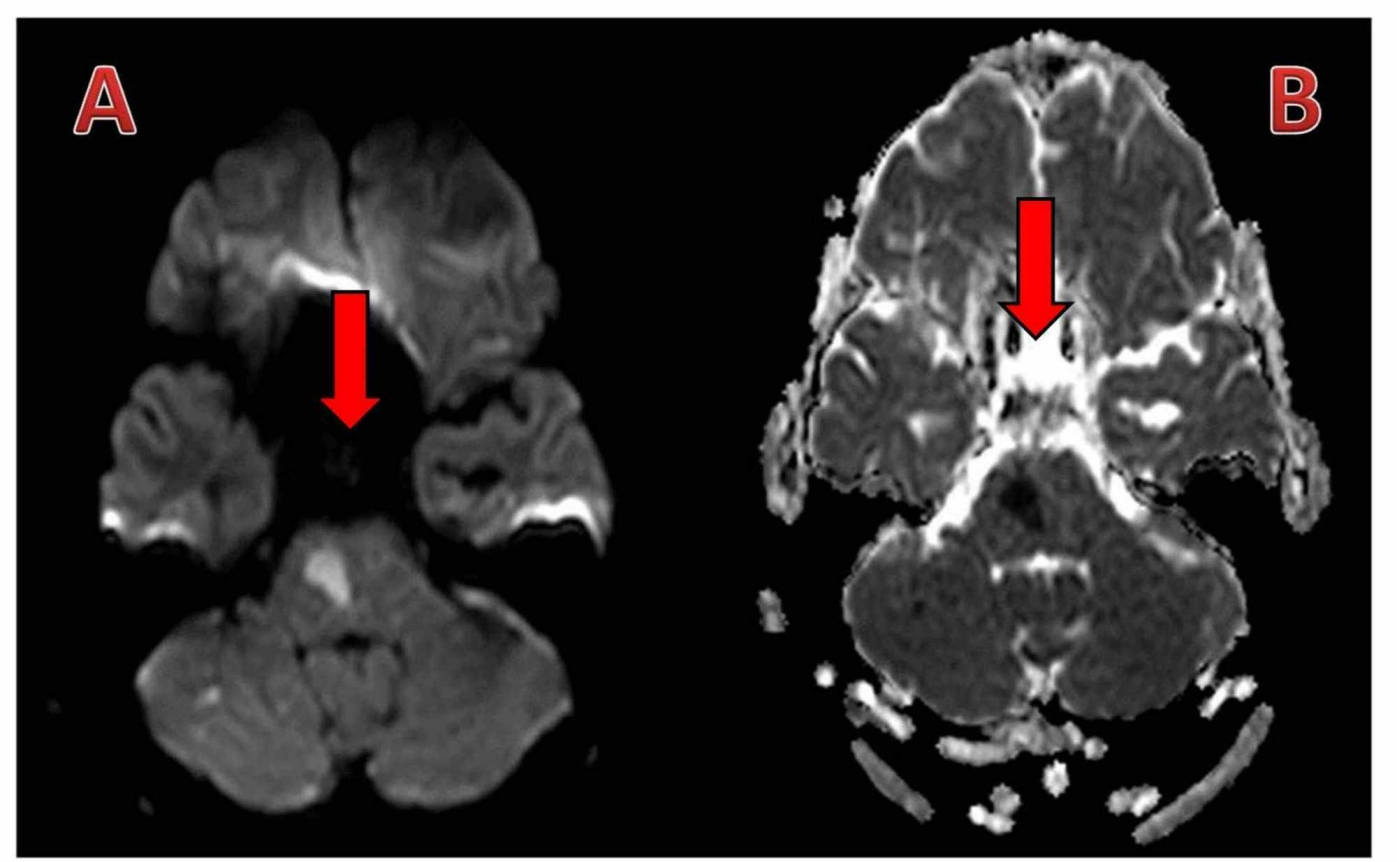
Diffusion weighted imaging (DWI) and apparent diffusion coefficient (ADC) of the brain (A) The acute lesion is a region of hypointensity in the paramedian pontine region. (B) DWI shows the relieved lesion a region of hyperintensity in the paramedian pontine region.
